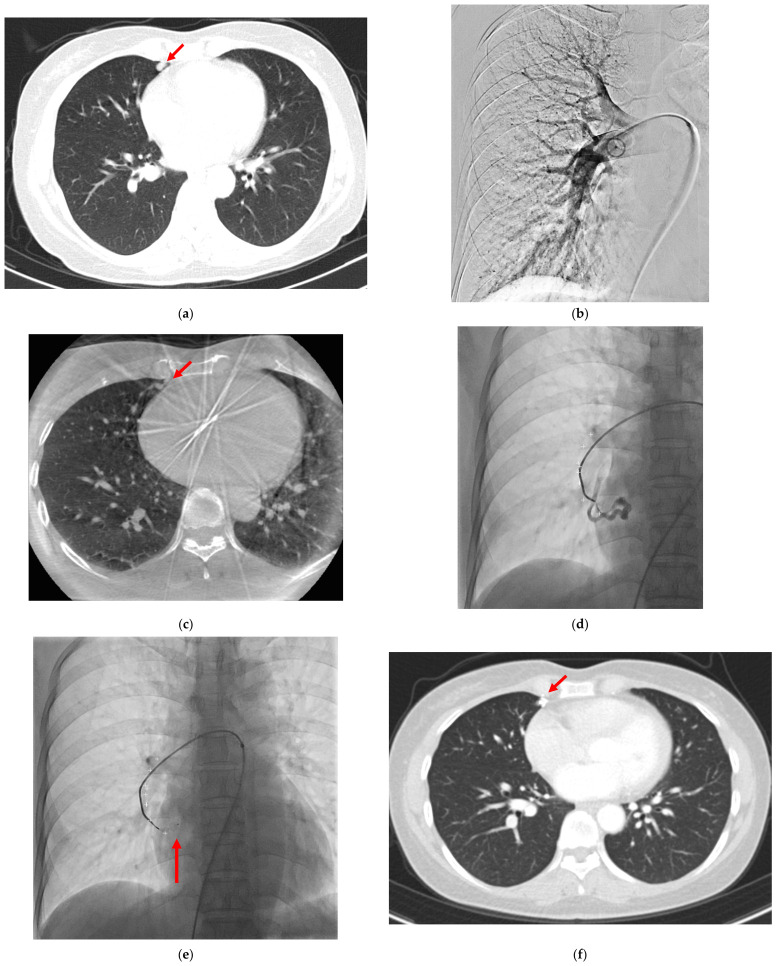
A 50-year-old woman with a pulmonary arteriovenous malformation (PAVM). (a) Preprocedural CT in the lung window reveals the nidus of the PAVM (arrow) in the right middle lobe; the lesion is obscured by the adjacent cardiac silhouette. (b) Digital subtraction angiography (DSA) from the right main pulmonary artery fails to clearly delineate the PAVM due to overlap with the heart shadow. (c) Noncontrast cone-beam CT (CBCT) localizes the PAVM (arrow) in the same position as on preprocedural CT. (d) Overlay fluoroscopy after CBCT demonstrates the course of the feeding artery from the right main pulmonary artery to the nidus. With the catheter advanced along this CBCT-derived trajectory, contrast injection clearly opacifies the PAVM. (e) Deployment of a vascular plug (arrow) achieves successful embolization. (f) Three-month follow-up CT confirms complete resolution of the PAVM with the vascular plug (arrow) in place.
